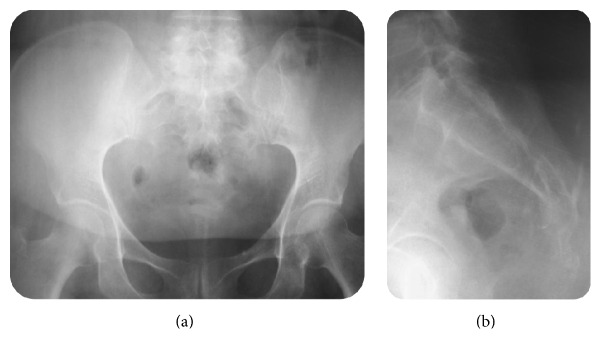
AP and oblique X-rays of pelvis showing fuzziness of the sacrococcygeal region with irregular margins.
